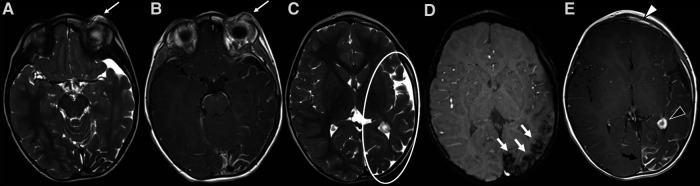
MRI of a 3-year-old child with Sturge-Weber syndrome and a capillary malformation (CM) on the left forehead, upper eyelid and periorbital region (V1 distribution). (A,B) On the left there is an increased thickness of the periorbital soft tissues (particularly of the skin and subcutaneous tissue) corresponding to the clinically visible mark, which shows a subtle hyperintensity on axial T2-weighted sequence and a faint but rather homogeneous enhancement on axial post contrast T1 weighted sequences (white arrows in A and B, respectively). (C–E) Typical associated intracranial features of SWS on the same side (left) of the CM, including atrophy of the cerebral hemisphere with enlargement of subarachnoid spaces (especially in the frontal, insular, parietal and occipital regions, white circle in C), cortical gyral hypointensities due to calcifications on SWI (white arrow in D), contrast enhancement of the leptomeningeal angioma and choroidal plexus hypertrophy on axial post-Gadolinium T1 weighted sequence (black arrow and arrowhead in E, respectively). A subtle enhancement of the left frontal bone is also evident (white arrowhead in E), due to the intra-osseus extension of the overlying CM.
